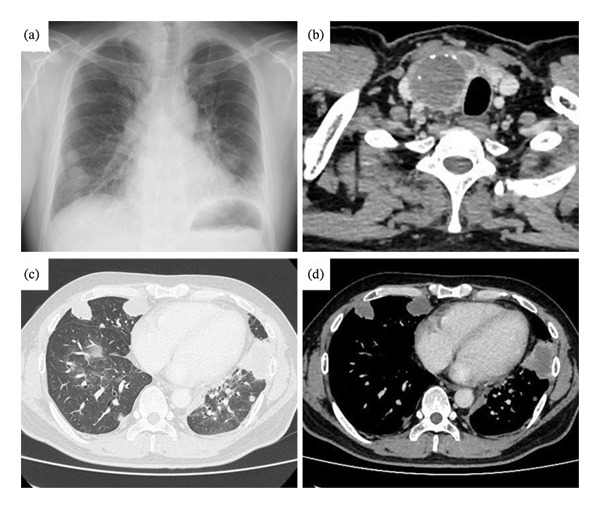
Chest imaging. (a) Chest X‐ray revealed multiple nodular shadows in both lower lungs. (b) Enhanced CT showed a 5‐cm tumor with poor contrast effect in the right lobe of the thyroid gland, compressing the right internal carotid artery and main bronchus. The tumor was accompanied by internal calcification and extensive contact with the capsular surface. (c) CT showed multiple mass lesions predominantly in both lower lungs. (d) Each lesion was accompanied by necrosis, as were the thyroid tumors.
